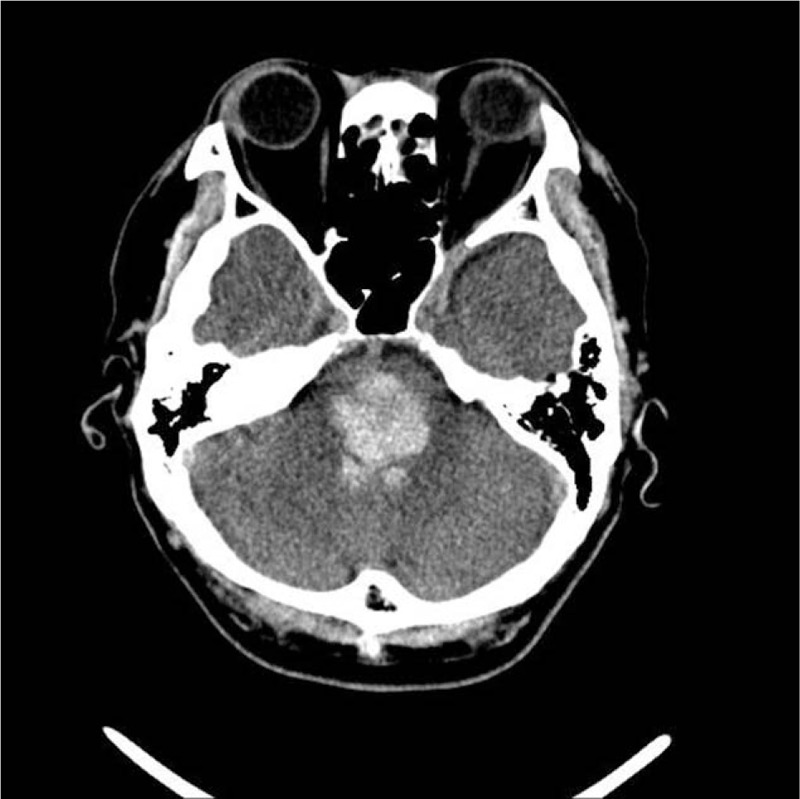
Computed tomography (CT) scan shows high attenuation in the acute pontine hemorrhage with intraventricular hemorrhage at the fourth ventricle.
